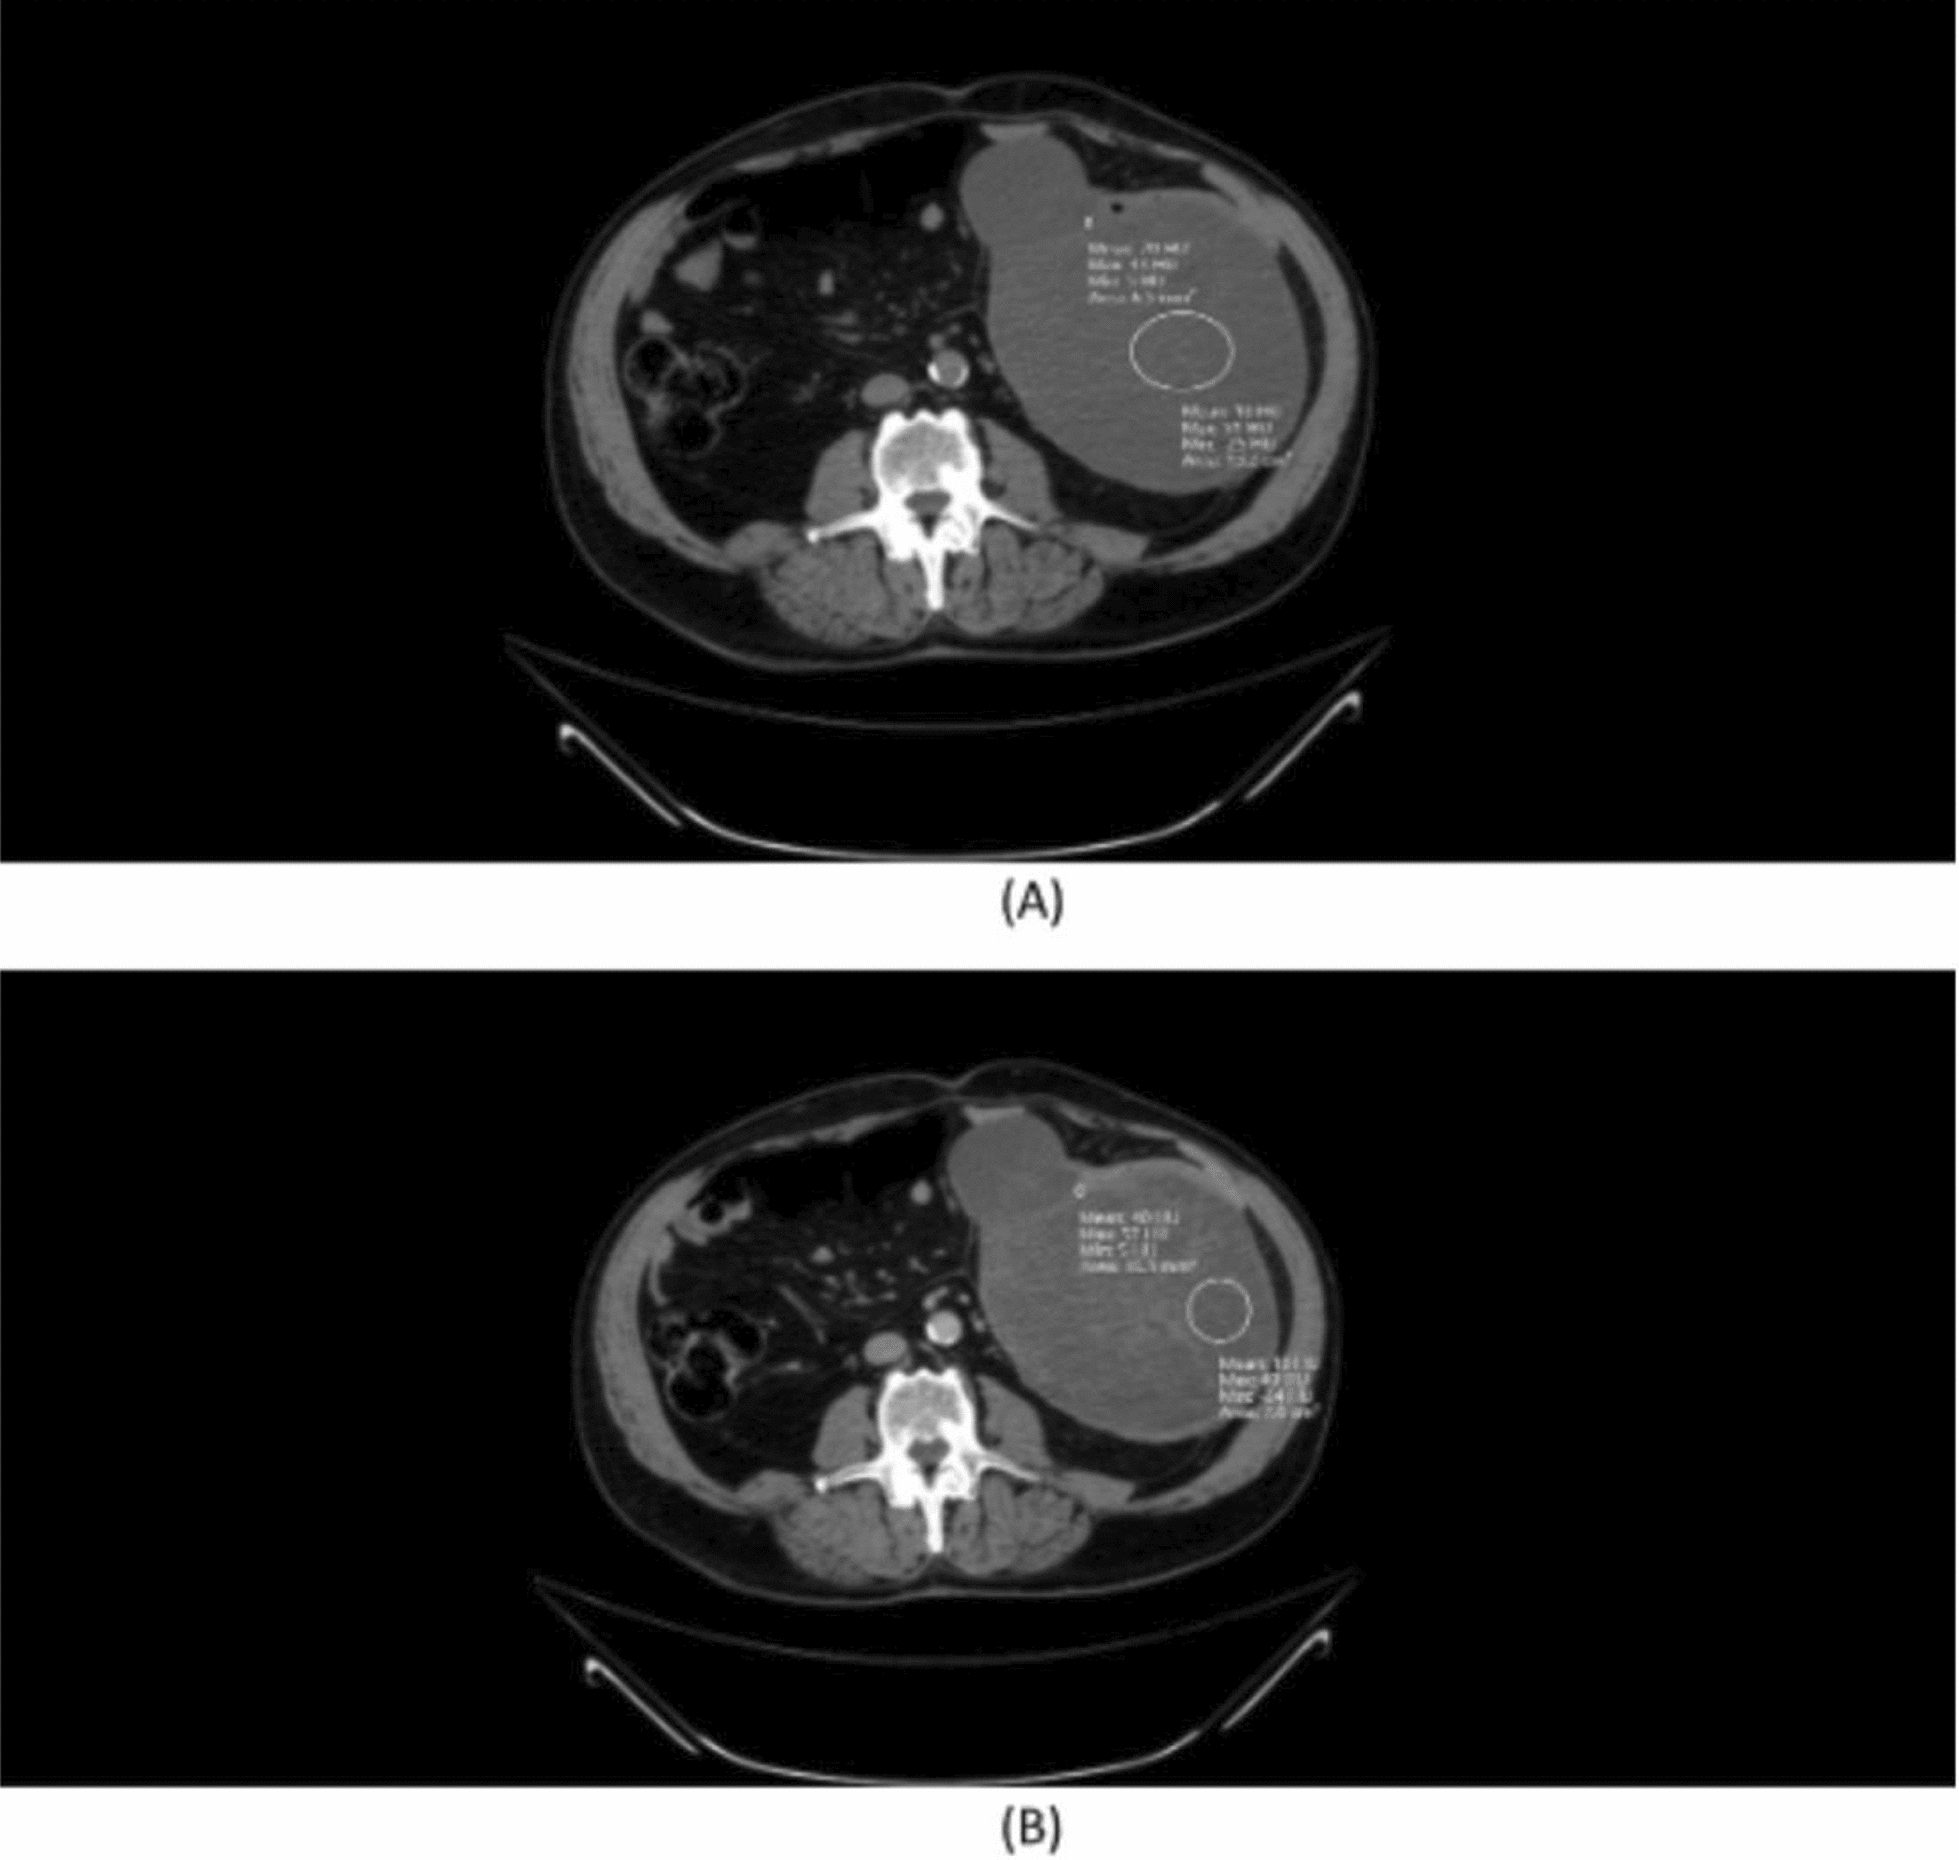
Axial abdominal computed tomography precontrast (A) and post contrast (B) showing density stability of the cystic portion (large circle with mean density of 10 Hounsfield units on both images)
